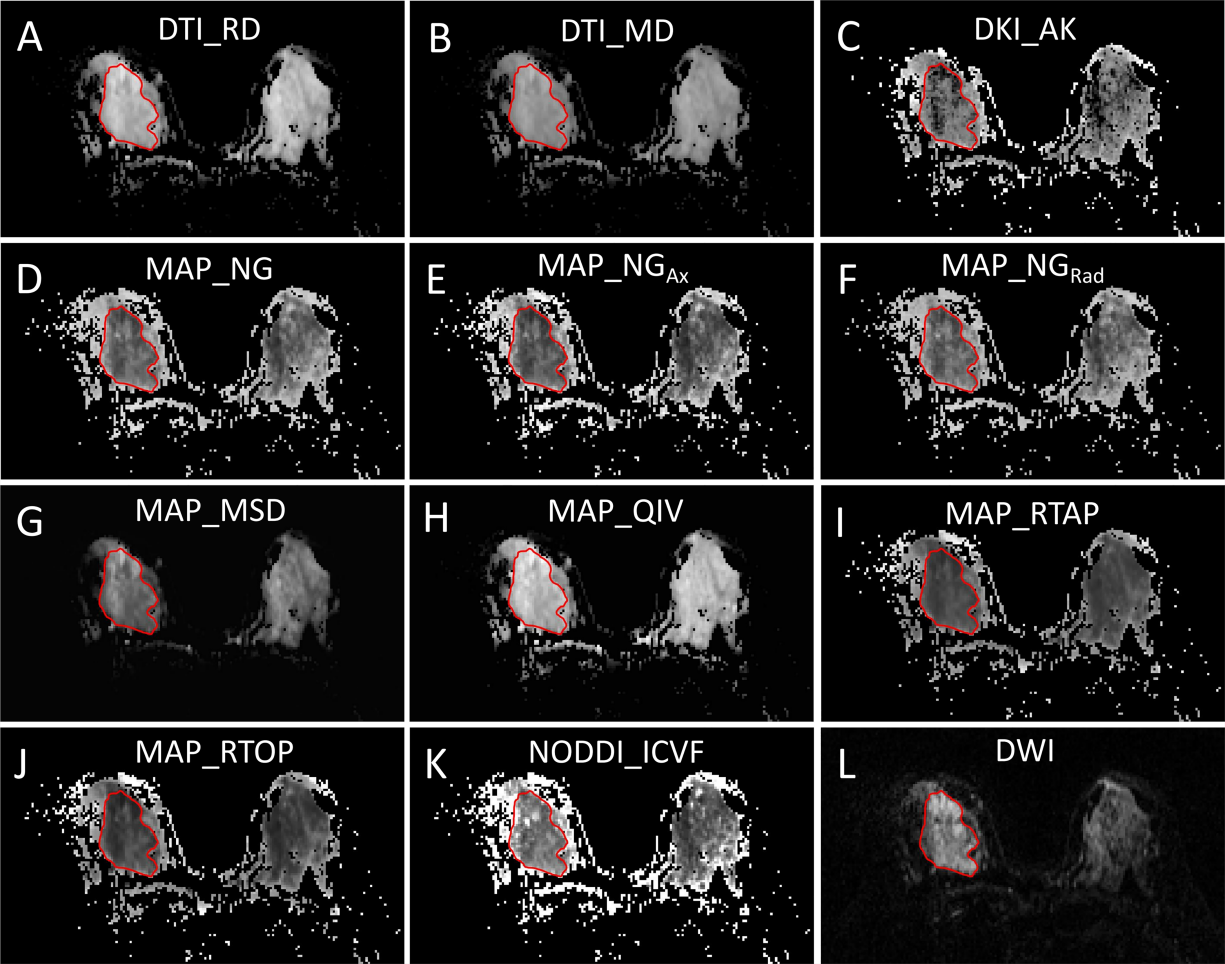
A 44-year-old woman with pathologically confirmed HER2-negative breast cancer in the right breast. (A–K) Quantitative DSI parameter measurements and (L) diffusion-weighted imaging (DWI) measurement.
